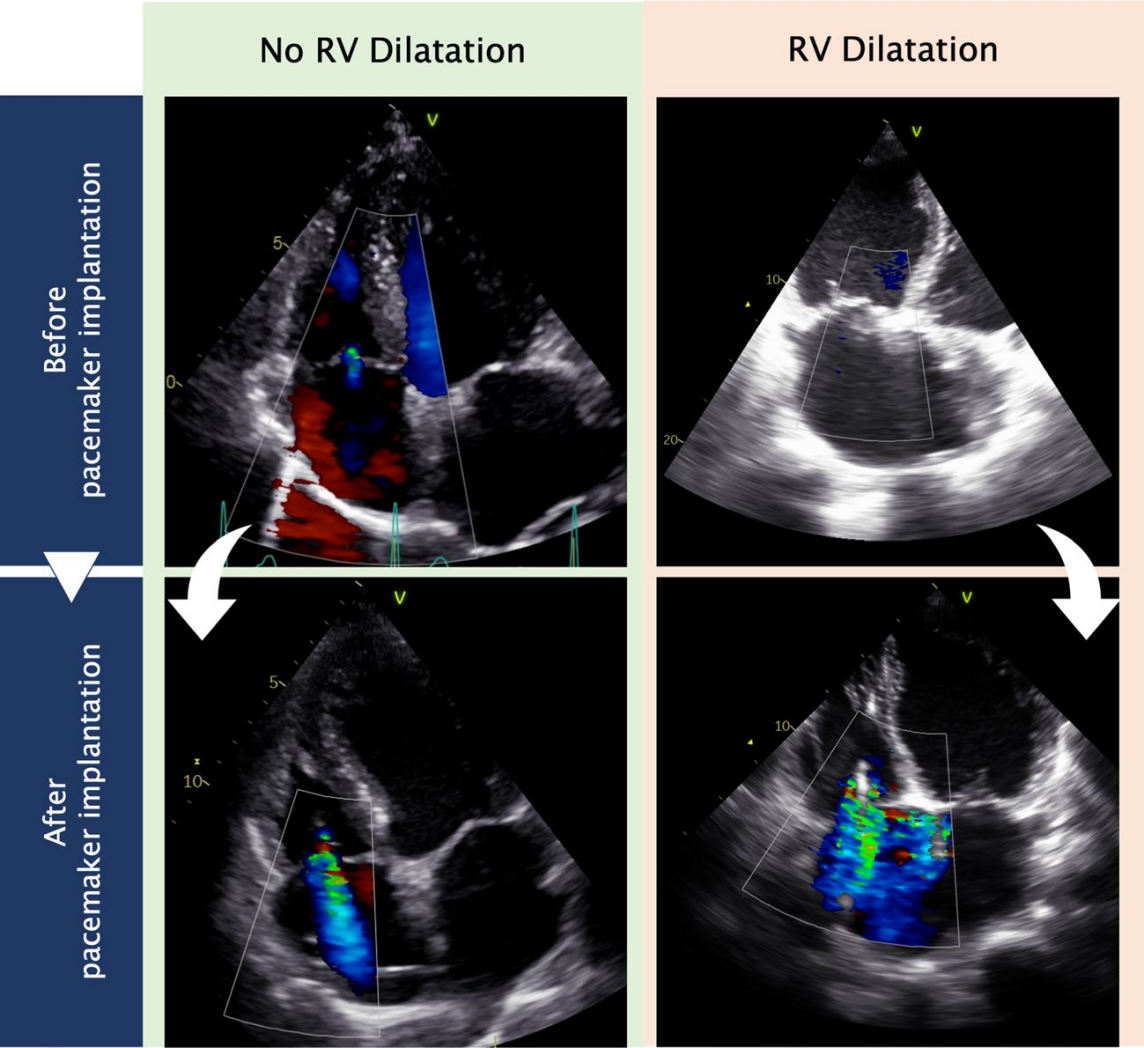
Echocardiography images evaluating tricuspid regurgitation before and after pacemaker implantation. Representative images from transthoracic echocardiography (4-chamber view) evaluating tricuspid regurgitation before and after pacemaker implantation in a patient without right ventricular dilatation (left column) and in a patient with right ventricular dilatation (right column)
